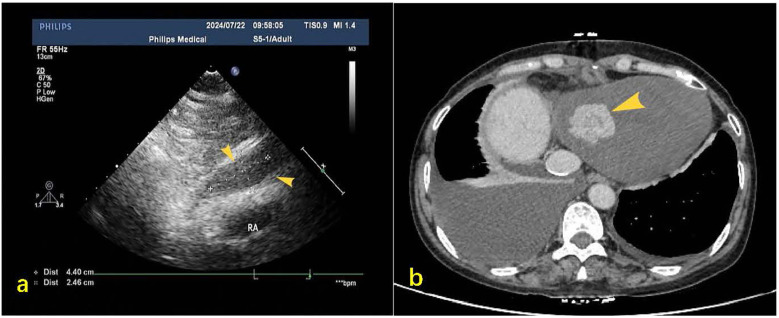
(a) Post-pericardiocentesis echocardiographic follow-up revealed a hypoechoic mass measuring approximately 44 × 25 mm in the pericardial cavity at the base of the right heart during subxiphoid examination (yellow arrow). (b) Contrast-enhanced CT on mediastinal window settings reveals an irregular soft tissue mass located at the base of the heart and the inferior aspect of the right atrium, measuring approximately 4.0 × 2.1 cm (indicated by the yellow arrow), exhibiting heterogeneous enhancement.
